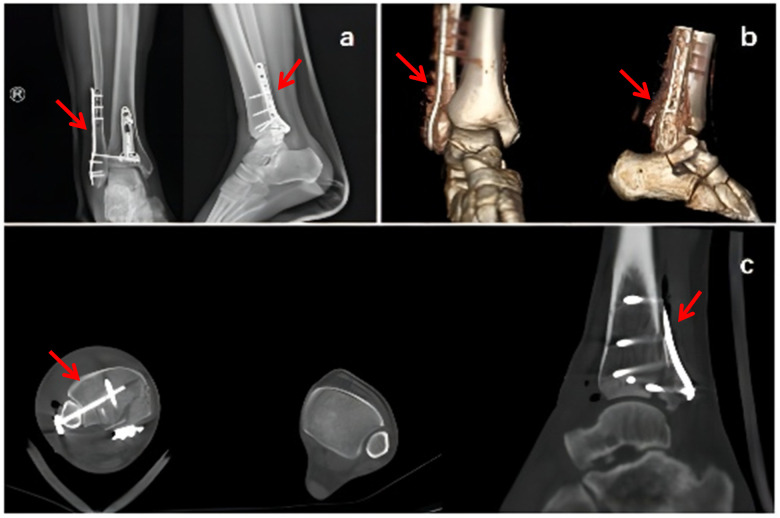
(a) The first review of the x-ray after operation indicates that the ankle is in place, and the alignment of the fracture can be aligned; (b, c) postoperative review of CT with three-dimensional reconstruction shows the Bosworth fracture-dislocation had been reset.
